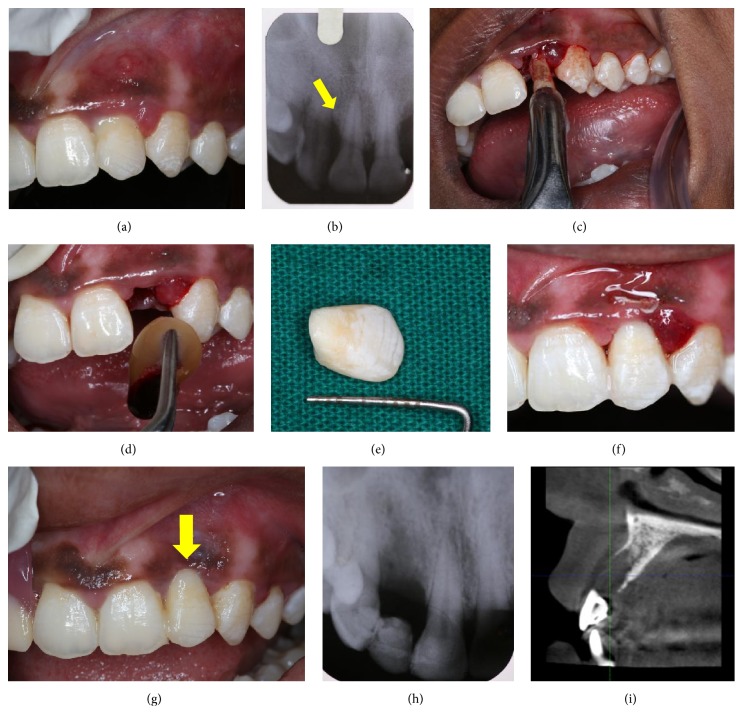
(a) Preoperative. (b) Preoperative radiograph. (c) Extraction of 22. (d) Insertion of PRF. (e) Customized NTP. (f) Immediate postoperative. (g) 1 week postoperatively. (h) 1 week postoperatively. (i) CBCT NTP with respect to 22.
